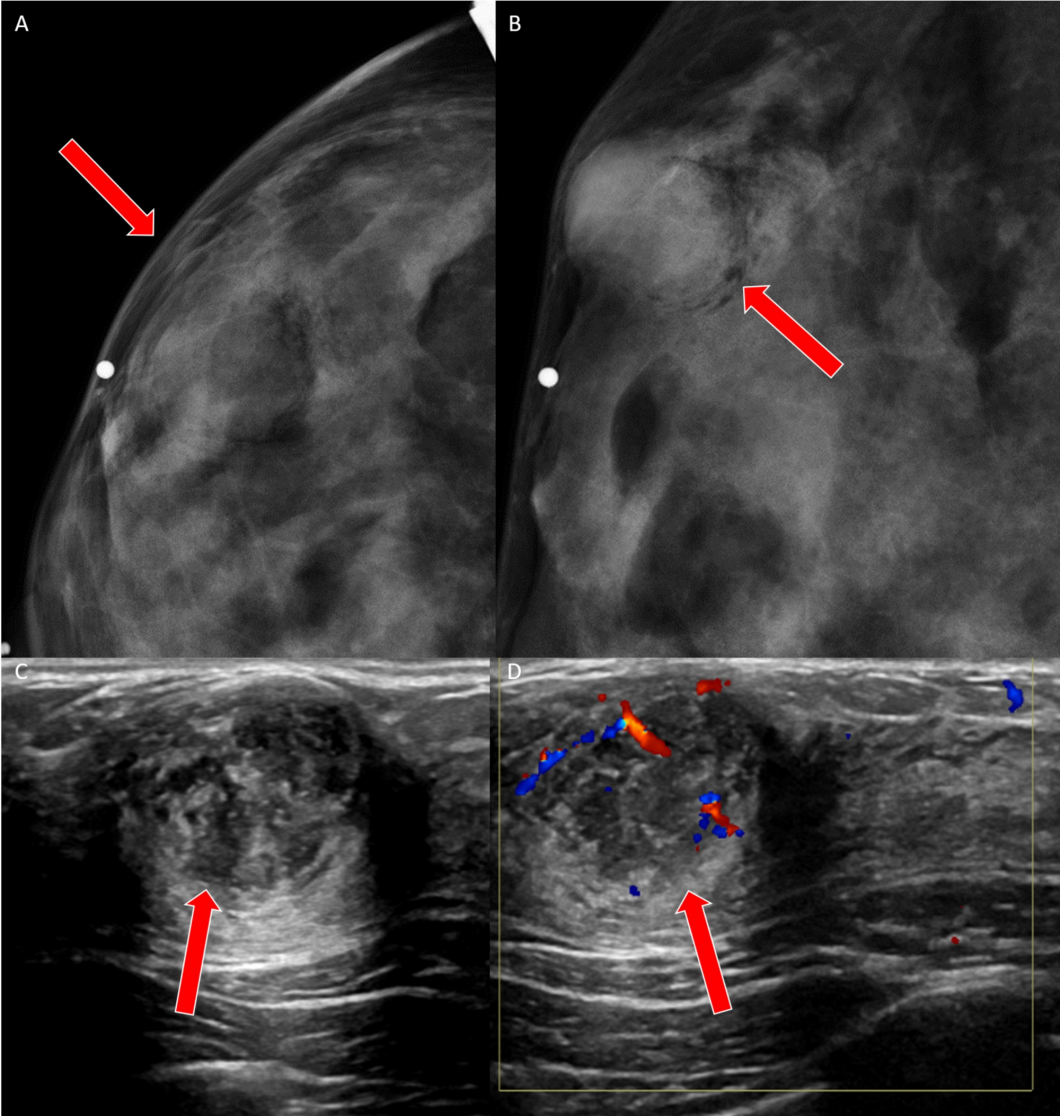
Case 4 imaging findings A, B: Right diagnostic mammogram shows a round circumscribed mass (red arrows) in the upper outer quadrant corresponding to the area of palpable concern. C, D: The ultrasound shows a round, circumscribed mass (red arrows) with heterogeneous echotexture and internal vascularity.
